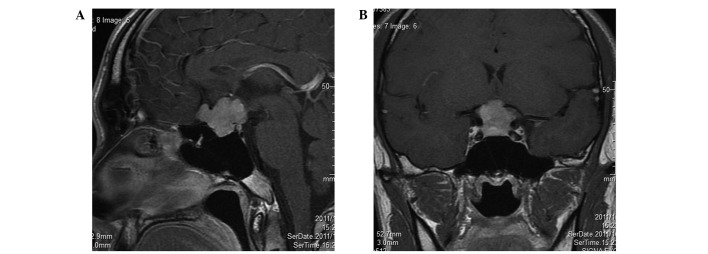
Tumor-enhanced magnetic resonance imaging (MRI) in the pituitary region showing heterogeneous contrast enhancement. (A) Tumor shadow in the sagittal view. (B) Tumor shadow in the coronal view.
